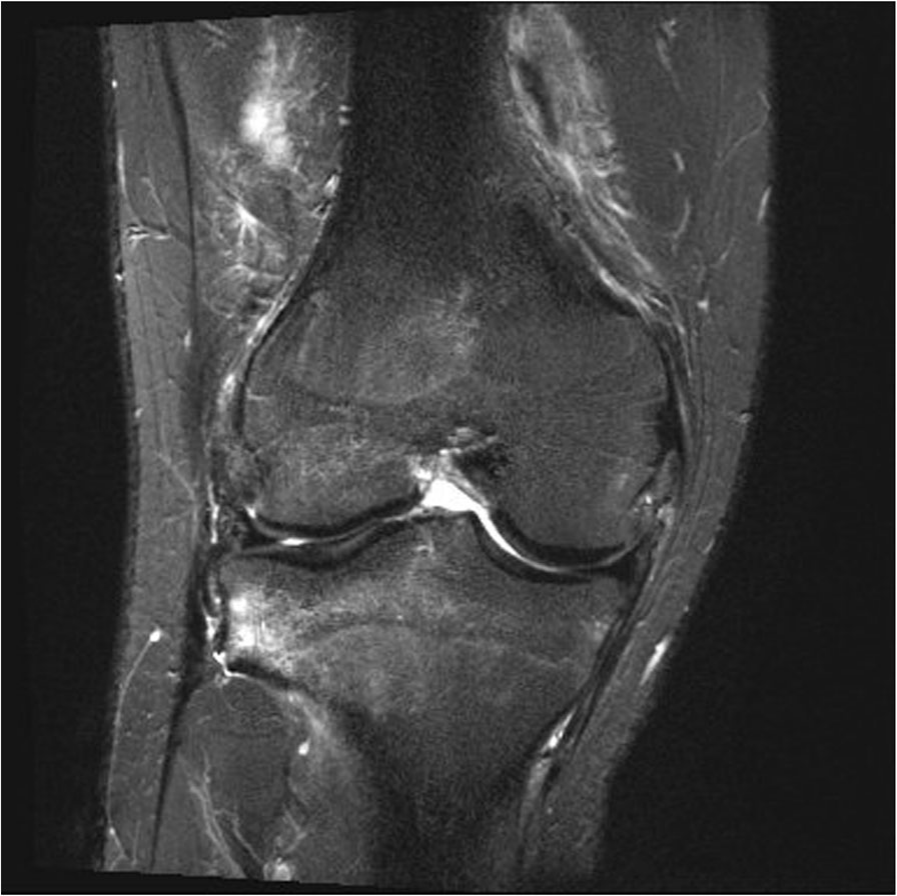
Coronal T2 MRI of the knee with fat saturation showing a ruptured ACL and high intensity signalling in the notch
